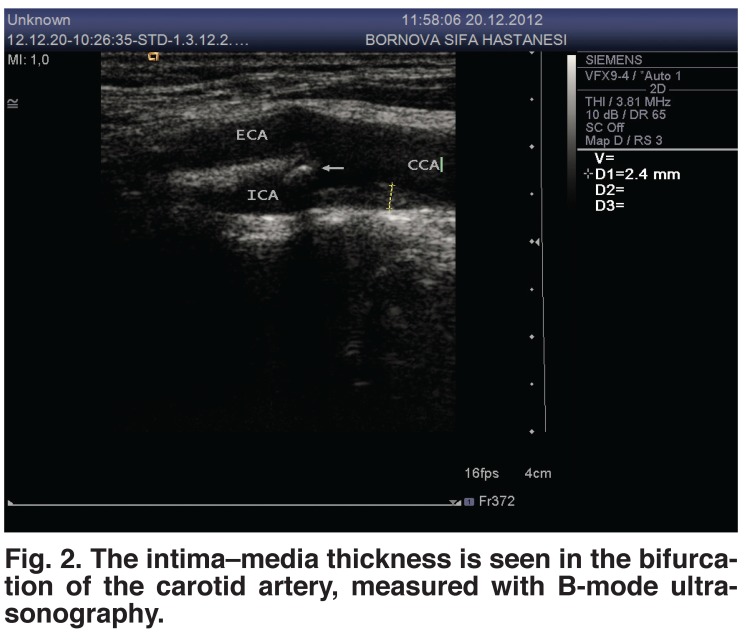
The intima–media thickness is seen in the bifurcation of the carotid artery, measured with B-mode ultrasonography.
